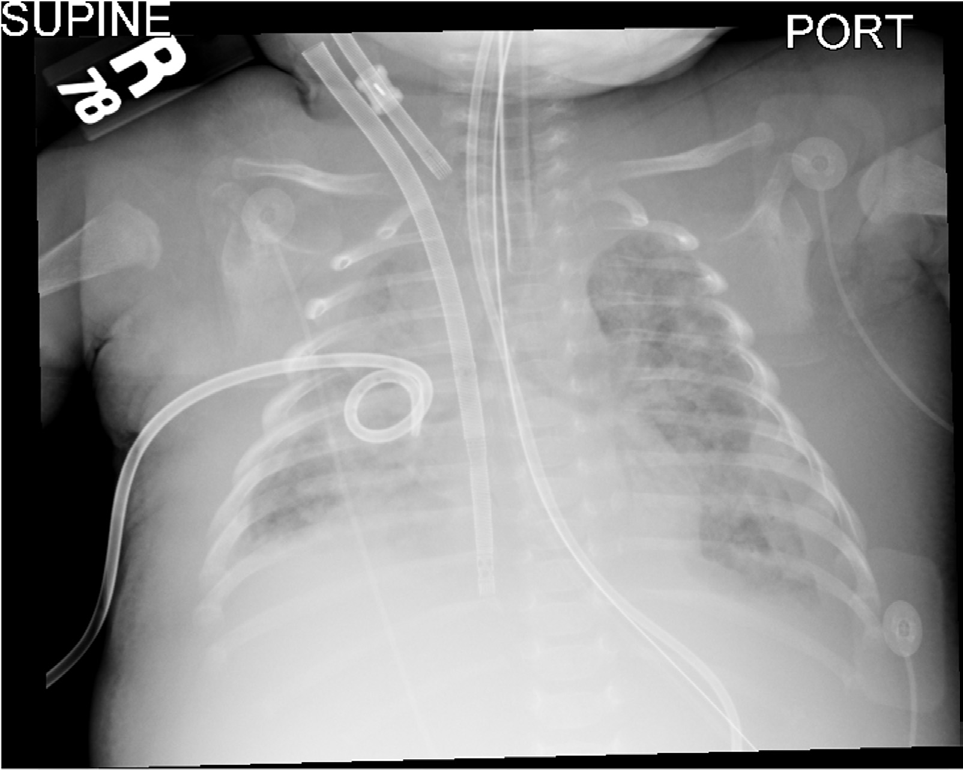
Chest radiographs 6 h after initiation of intrapulmonary percussive ventilator therapy. There was improvement of aeration of the left lung with persistent hazy opacifications in the right upper and middle lobes.
